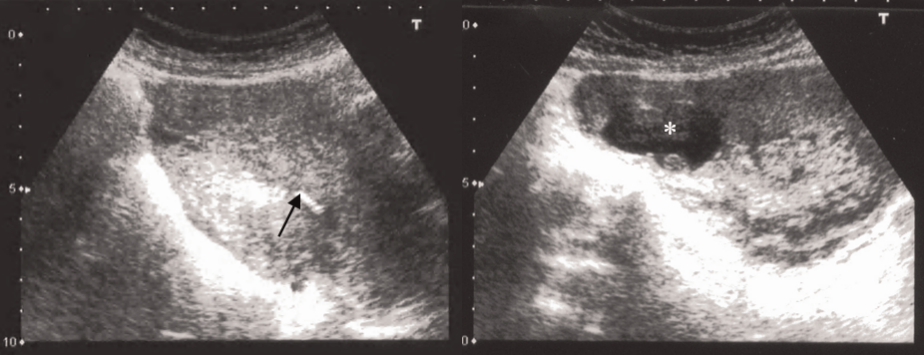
Transabdominal ultrasound of the uterus after evacuation of the non-viable intrauterine pregnancy. The image in the left panel is a sagittal section showing no retained products of conception after the uterine evacuation (black arrow). The image in the right panel is a transverse section showing the persistence of the right cornual gestational sac (*) in continuity with the uterine cavity.
